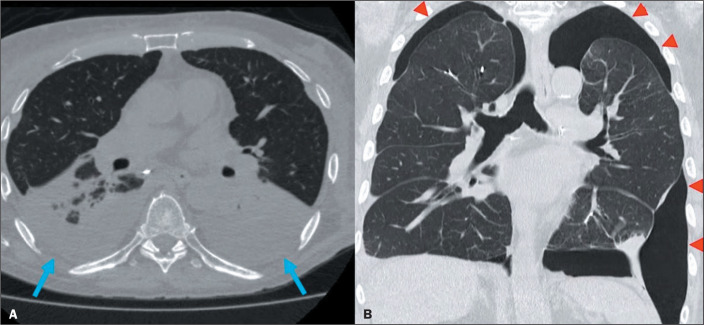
CT scans showing acute pleural complications after LT. A: Moderate
